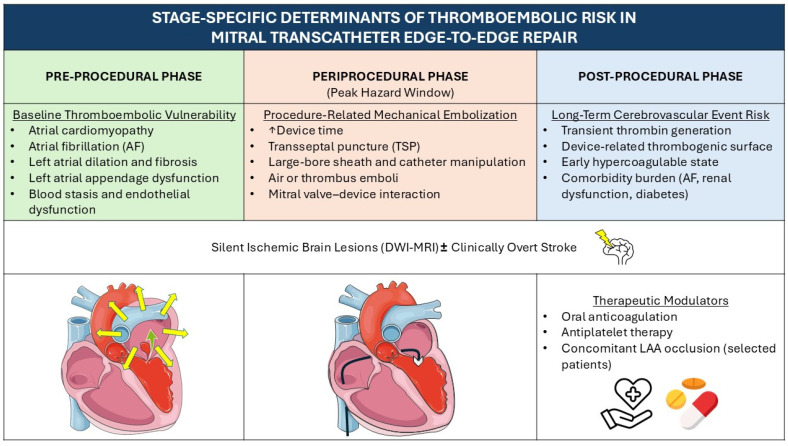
Stage-specific determinants of thromboembolic risk in mitral transcatheter edge-to-edge repair. Abbreviations: AF: atrial fibrillation; DWI-MRI: diffusion-weighted magnetic resonance imaging; LAA: left atrial appendage; TSP: transseptal puncture.
